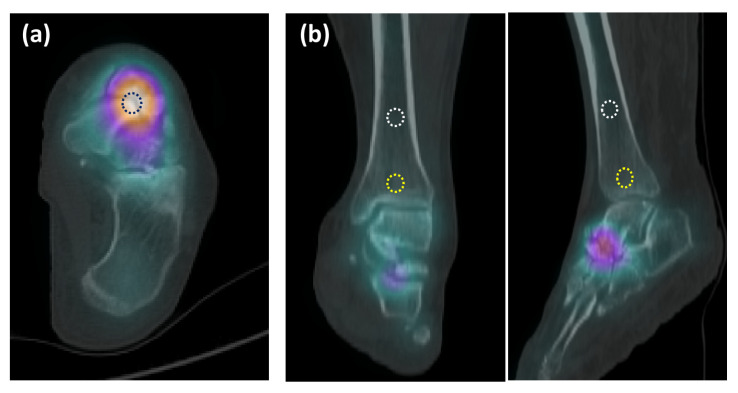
VOI was delineated on SPECT/CT images in MWS cases. (a) VOI on the lateral aspect of navicular bone presented as a dashed black circle; (b) a dashed yellow circle and the white circle signifies the VOIs on the tibial metaphysis (Tm) and tibial diaphysis (Td), positioned at the distal tibia, respectively. The color changes at TN joint in SPECT/CT indicate high radiotracer uptake.
